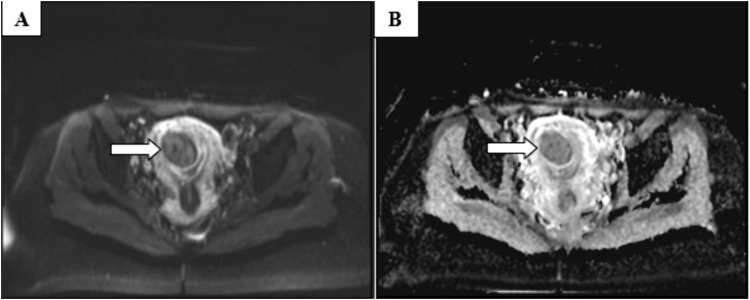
Pelvic MRI of a 35-year-old female performed at MNH, showing DWI and ADC sequences (A &B) respectively.
